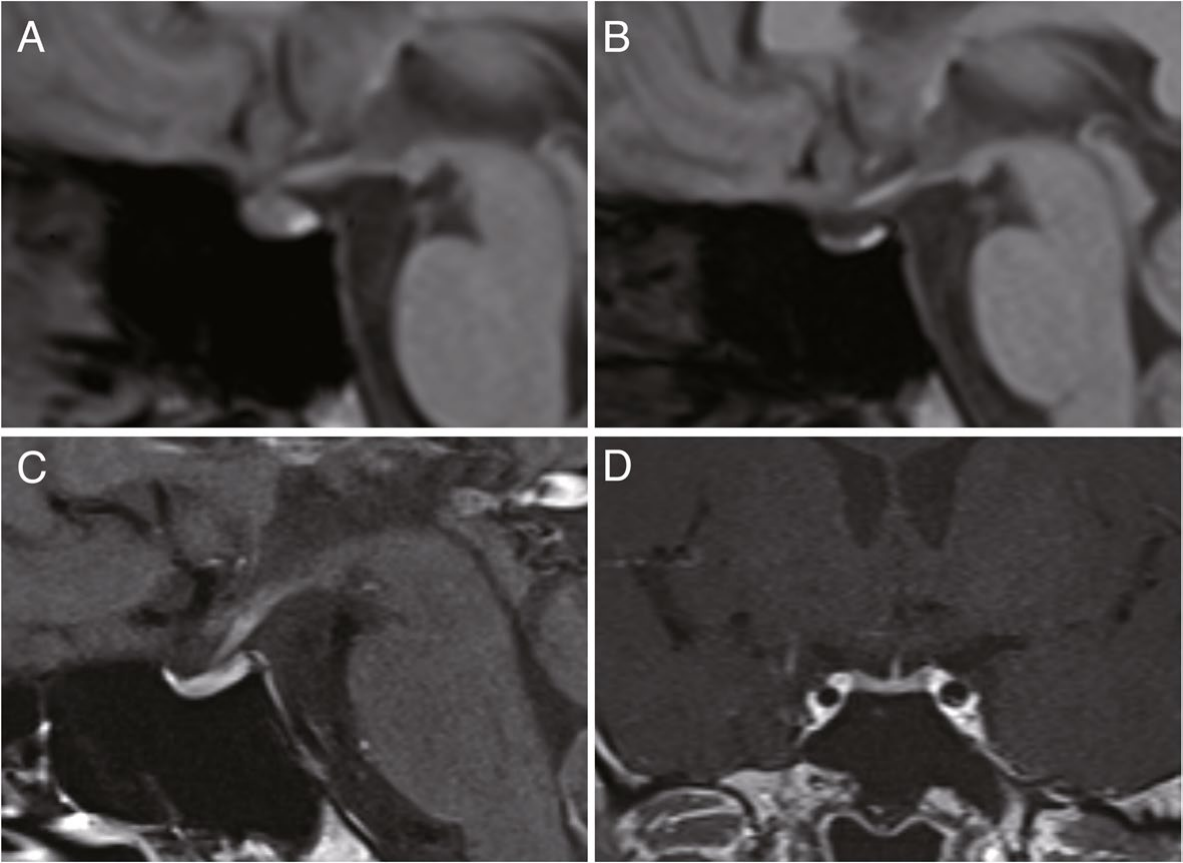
A T1-weighed pituitary MRI performed around two years before the onset. B T1-weighed pituitary MRI performed around two weeks after the replacement with hydrocortisone. C, D T1-weighed Gadolinium-enhanced pituitary MRI performed one month after the onset
